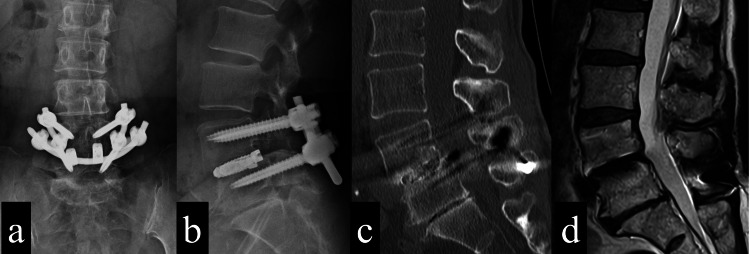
Imaging findings two years after surgery 4a: A plain AP X-ray image of the lumbosacral spine shows no adjacent segmental disorders. 4b: A plain lateral X-ray image of the lumbosacral spine shows no adjacent segmental disorders. 4c: CT sagittal view (midline) shows intervertebral disc height was not changed compared to preoperative height. 4d: MRI sagittal view (midline) shows no canal stenosis.
